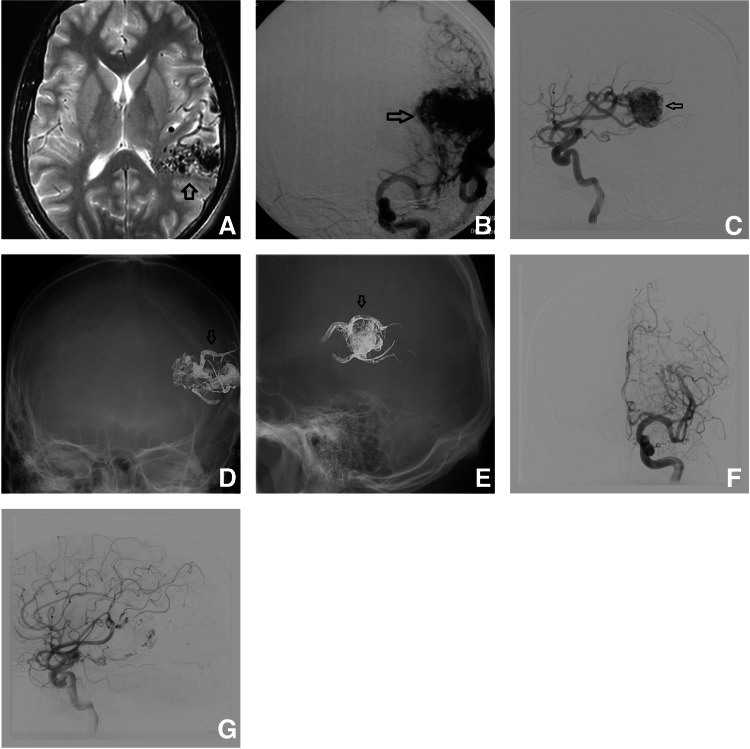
MRI shows an angular cortex compact nidus pointed at by an arrow (A), anteroposterior (B), and lateral (C) views of pre-embolization DSA shown by an arrow. (D) and (E) show anteroposterior and lateral views of Onyx (EVOH-based liquid embolizing agent) cast respectively marked by an arrow. Immediate post-embolization DSA shows in the anteroposterior (F) and lateral (G) views, showing complete obliteration of the AVM. Follow-up DSA after two years (not shown here) showed similar results. AVM - arteriovenous malformation; DSA - digital subtraction angiogram
